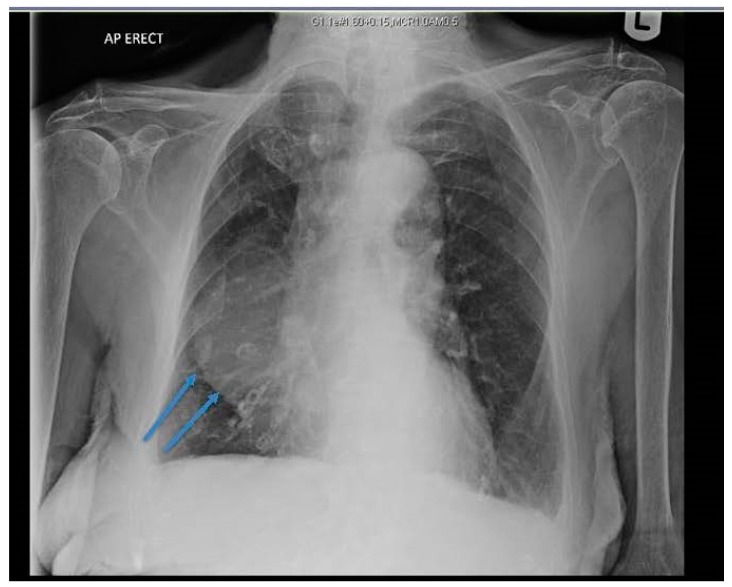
Chest X-ray (CXR) showing right sided soft tissue density, hemispherical in shape, projected over the right middle and lower zones (arrows).
